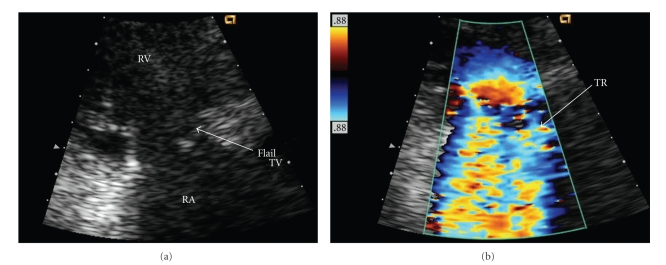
(a) Transthoracic 2D echo parasternal long-axis systolic view of a flail anterior tricuspid valve leaflet (TV). Right atrium (RA) and right ventricle (RV) are labeled. The tricuspid valve annulus is not dilated, measuring 3 cm in maximum diameter. (b) Color flow Doppler demonstrating severe tricuspid regurgitation (TR).
